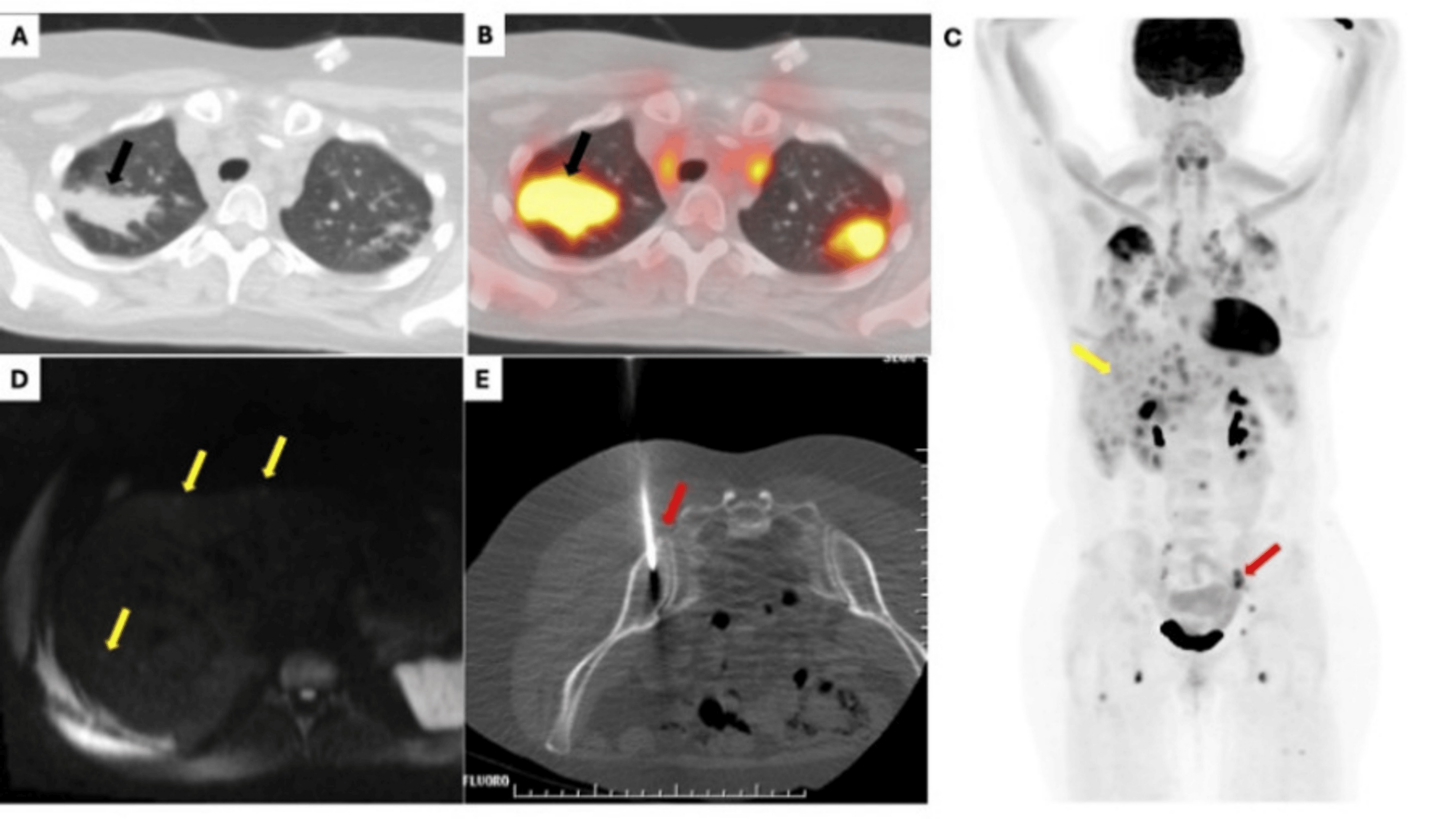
Imaging findings Axial CT (A) of the chest shows consolidative opacity (black arrow) within the right upper lung lobe, which demonstrates high FDG (black arrow) uptake on the corresponding PET (B). PET Maximum intensity projection image (C) demonstrates multiple diffusely distributed foci of FDG avidity in the chest, abdomen, and pelvis. The FDG foci in the liver (yellow arrow) correspond to the foci of diffusion restriction (yellow arrow) on diffusion-weighted images (D). The FDG-avid left iliac lesion (red arrow) was later biopsied for tissue sampling under CT guidance (E) CT: computed tomography; FDG: fluorodeoxyglucose; PET: positron emission tomography
